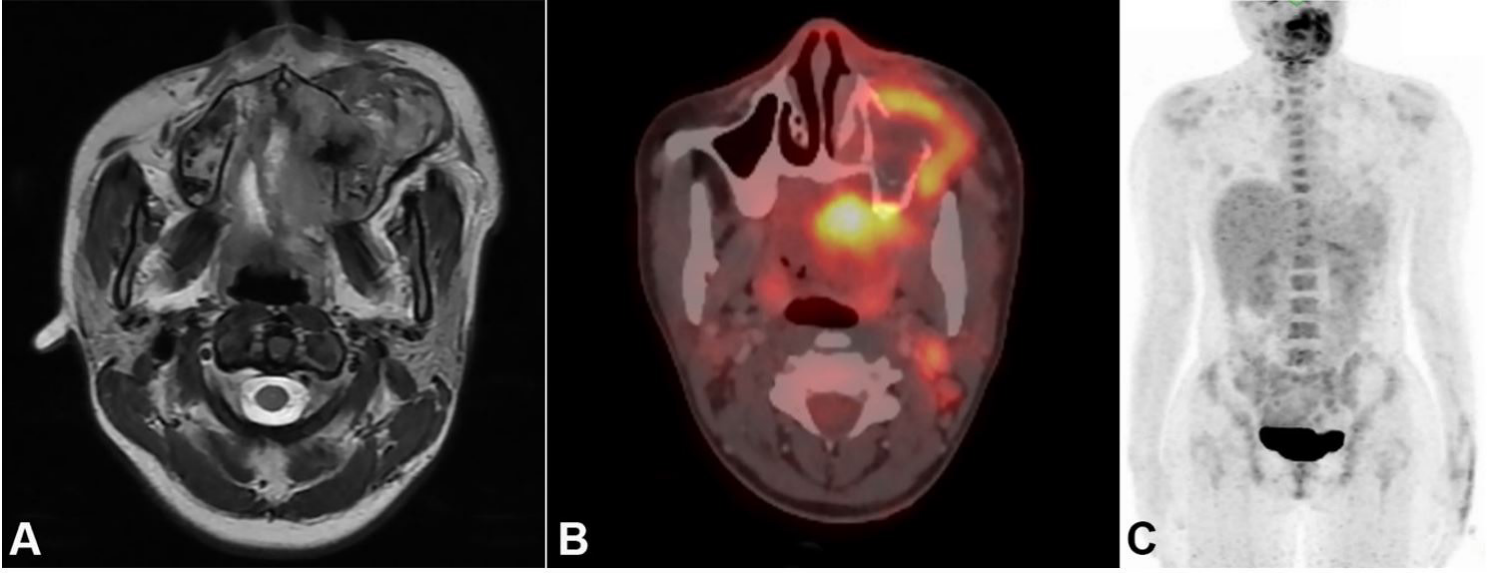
A – MRI T2 weighted image displaying the tumor extension; B – PET-CT scan demonstrating a fluorodeoxyglucose (FDG) avid tumor; C – Whole body positron emission tomography-computed tomography (PET-CT) scan without distant metastases.
